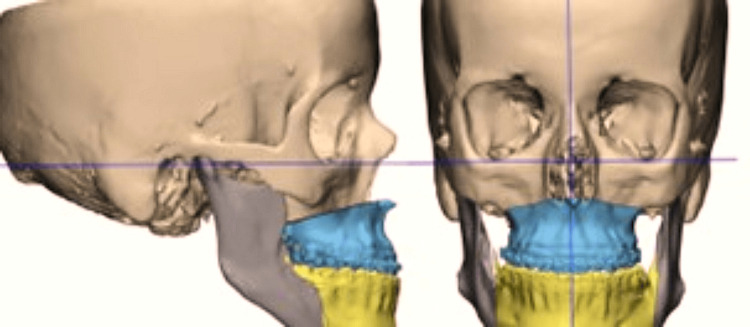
Virtual planning of the osteotomy after 3D reconstruction of a full skull CT scan.
